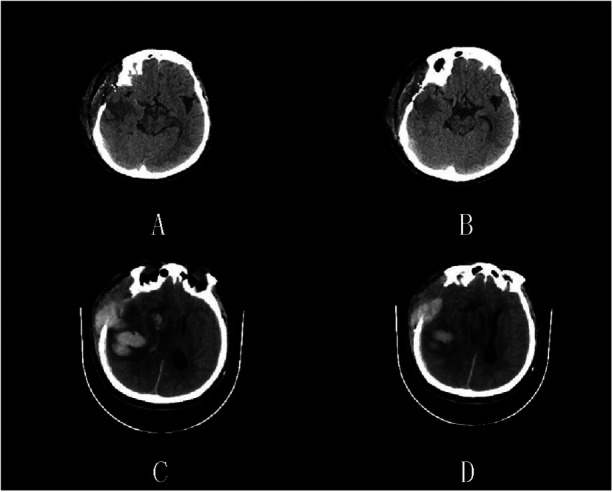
On the 7th postoperative day of case 2, the brain CT images were re examined. (A,B) Brain CT examination on the 7th day after surgery showed complete removal of intracerebral hematoma in the right temporal lobe. (C,D) The patient's brain CT emergency examination showed an intracerebral hematoma in the right temporal lobe.
